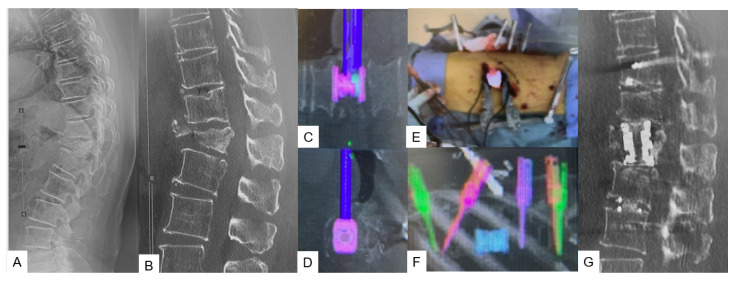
Case 1, 83-year-old female, T12 osteoporotic vertebral fracture, T12 MIS corpectomy. (A) Preoperative lateral spine radiogram, (B) Preoperative sagittal reconstruction CT, (C) Intraoperative coronal navigation image, (D) Intraoperative axial navigation image, (E) Intraoperative image, (F) Intraoperative pedicle screws and cage navigation image, (G) Postoperative sagittal reconstruction CT.
